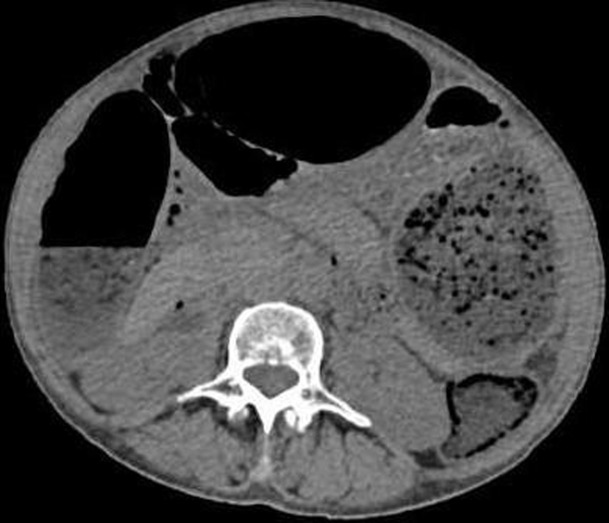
abdomino-pelvic CT scan in axial view, showing distension of the stomach and intestines; the distended large bowel anterior to the liver displaced it medially, suggestive of a long-standing process
